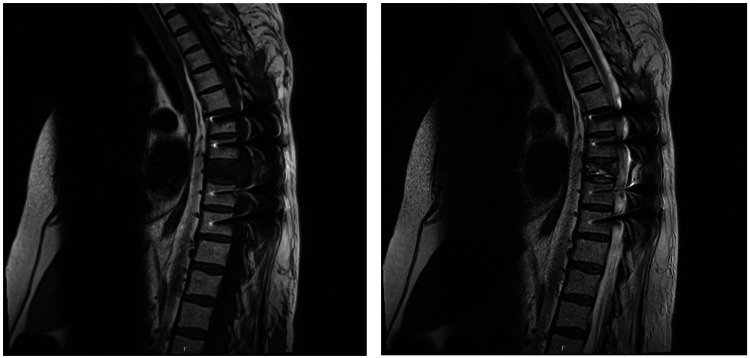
Magnetic resonance imaging (MRI) of the dorsal spine after surgery for the decompression of the spinal cord, exhibiting the removal of the tumor lesion along with part of T8 and the fixation of the remaining spine.
